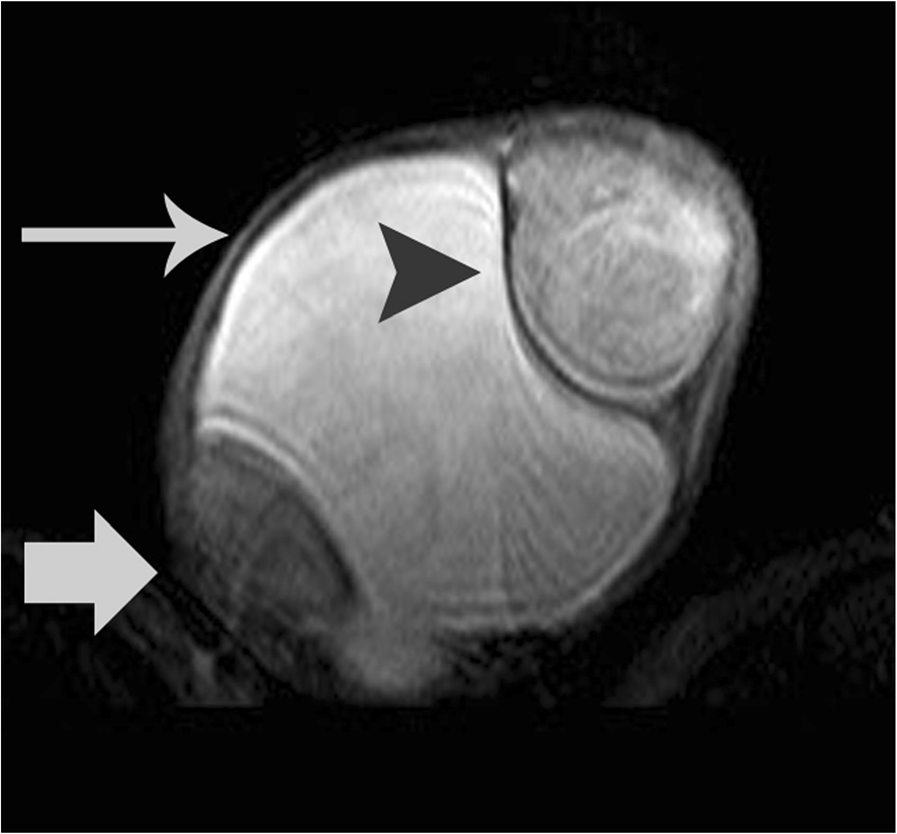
Transverse T2-weighted image demonstrates left paratesticular tumor heterogeneity. The mass (*arrowhead*) was slightly hyperintense when compared to the ipsilateral testicular parenchyma (*arrow*). Left hydrocele (*long arrow*)
